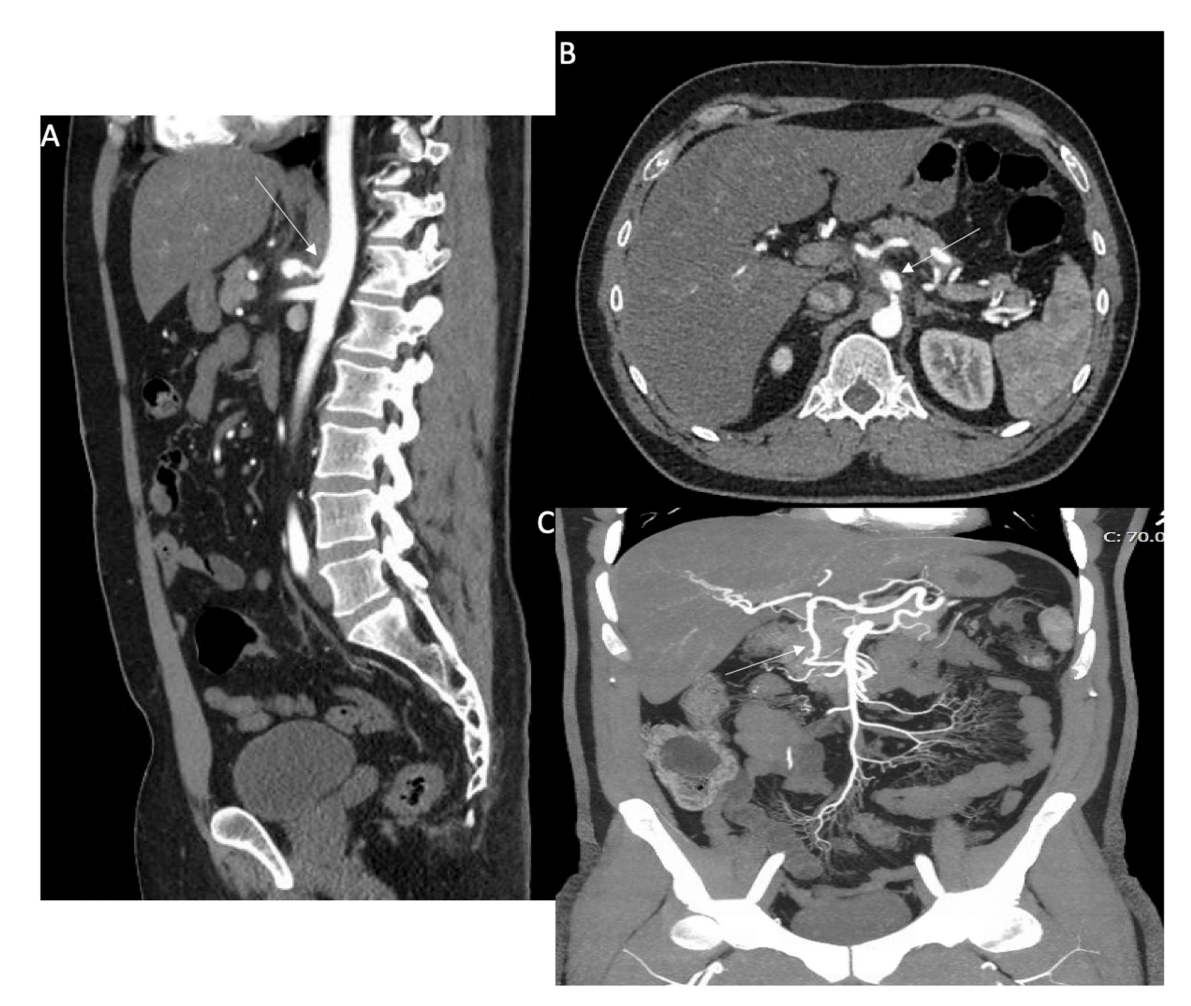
CTA of the abdomen and pelvis. A) Sagittal view shows compression of the celiac artery by the median arcuate ligament (white arrow).
